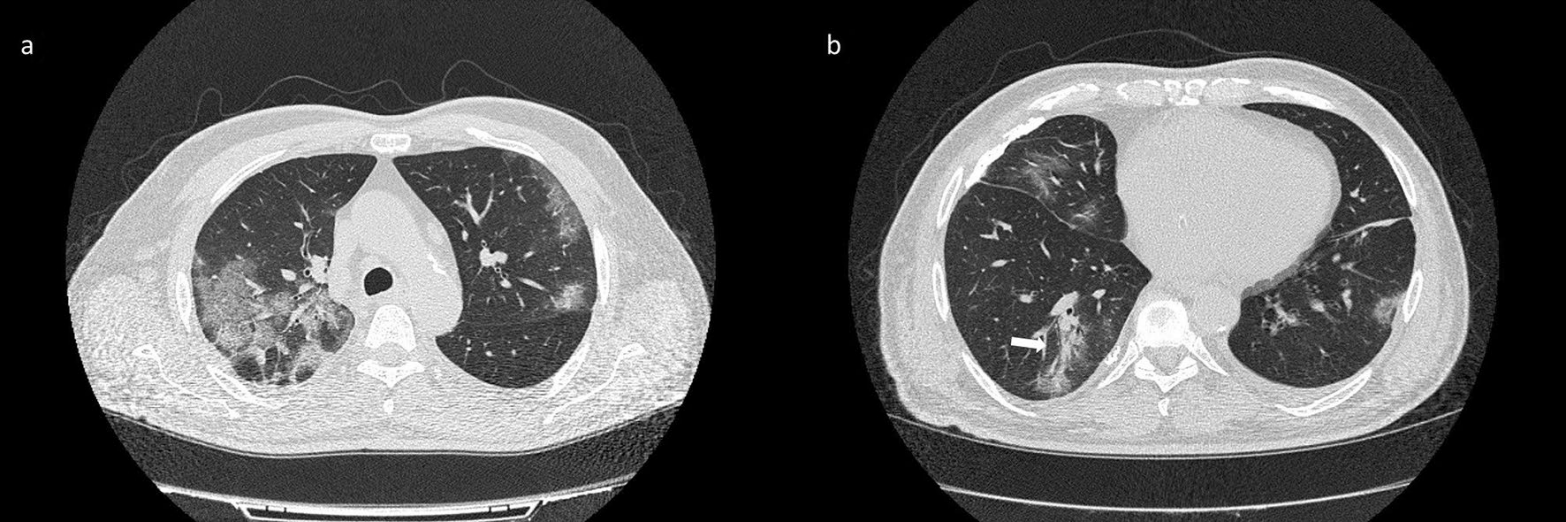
(a) CT scan through upper lobes shows reticular pattern superimposed on the background of GGO with patchy distribution identifying a crazy paving pattern; (b) lower CT scan shows multifocal GGOs and consolidation with air bronchogram in the right lower lobe (withe arrow).
